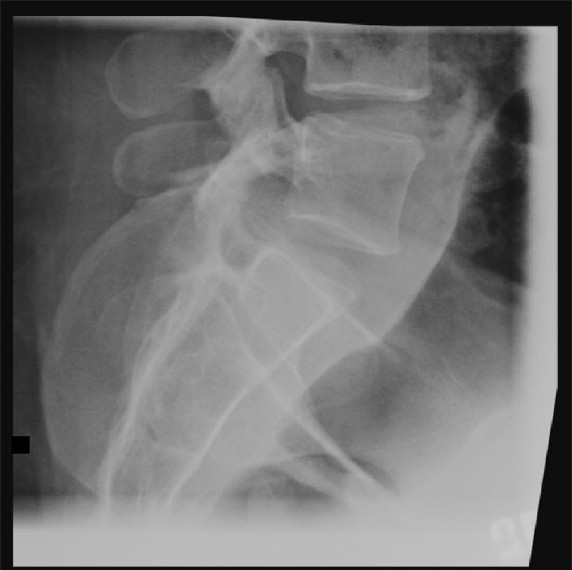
Plain lateral radiograph of the lumbar spine, demonstrating subtle widening of one of the L5–S1 neuroforamina
